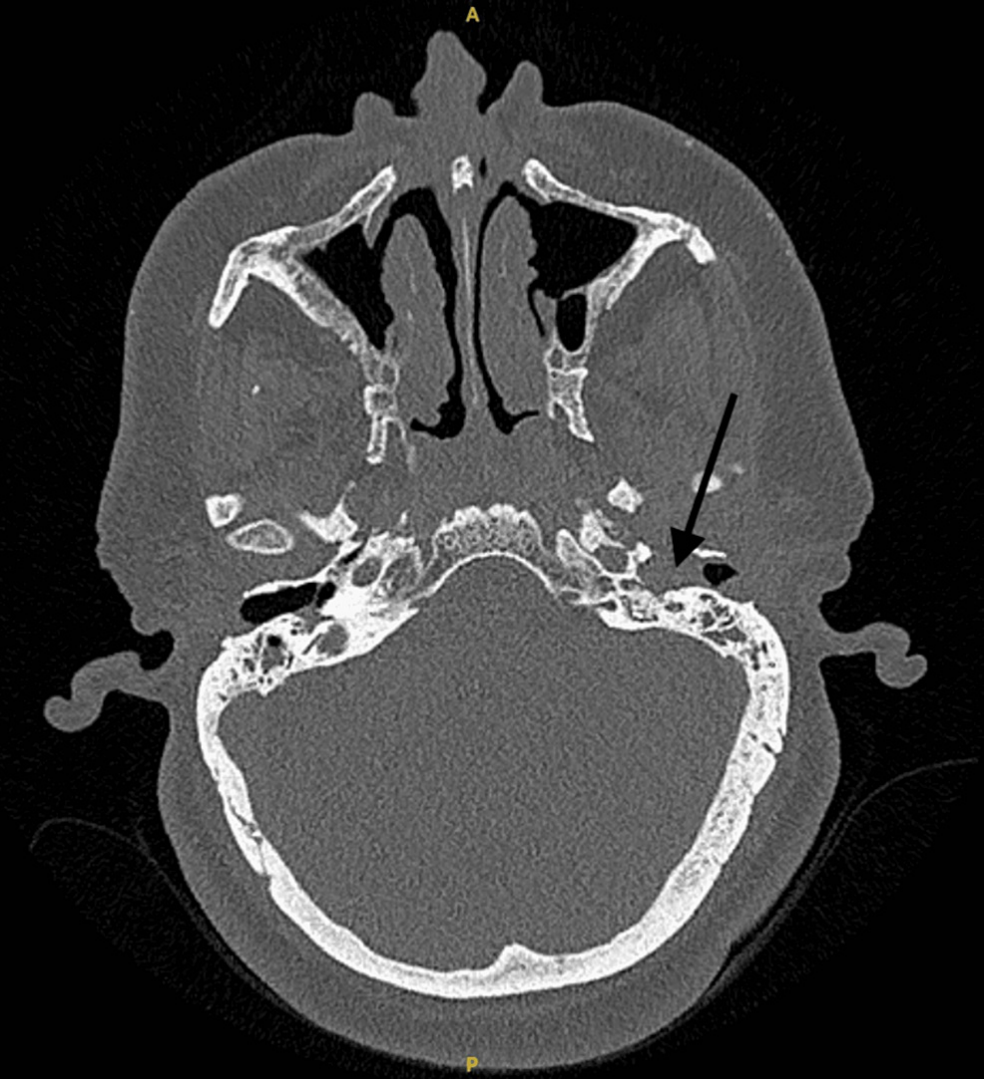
Axial CT scan showing erosion of left EAC and left EAC polyp (indicated by an arrow). EAC: External auditory canal
